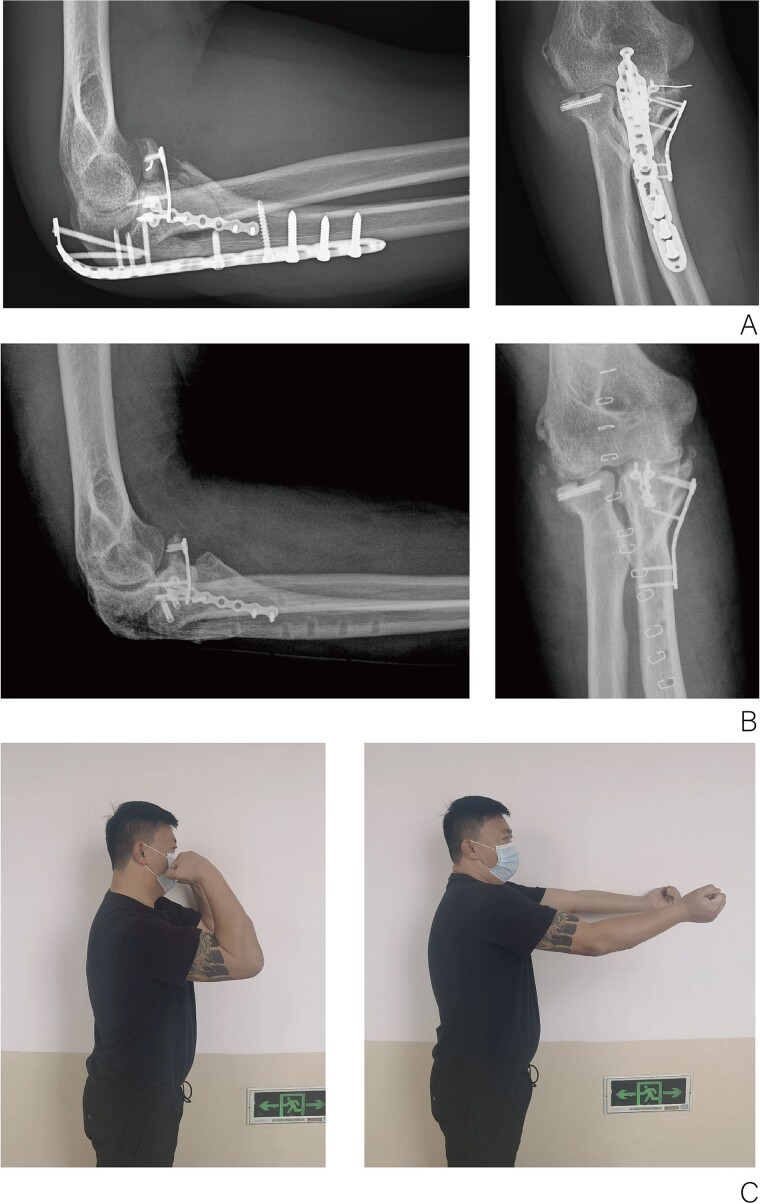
(A) X-ray of elbow at 3 months after surgery. (B) X-ray of elbow at 1 year after surgery. (C) Function of elbow at 6 months after surgery.
